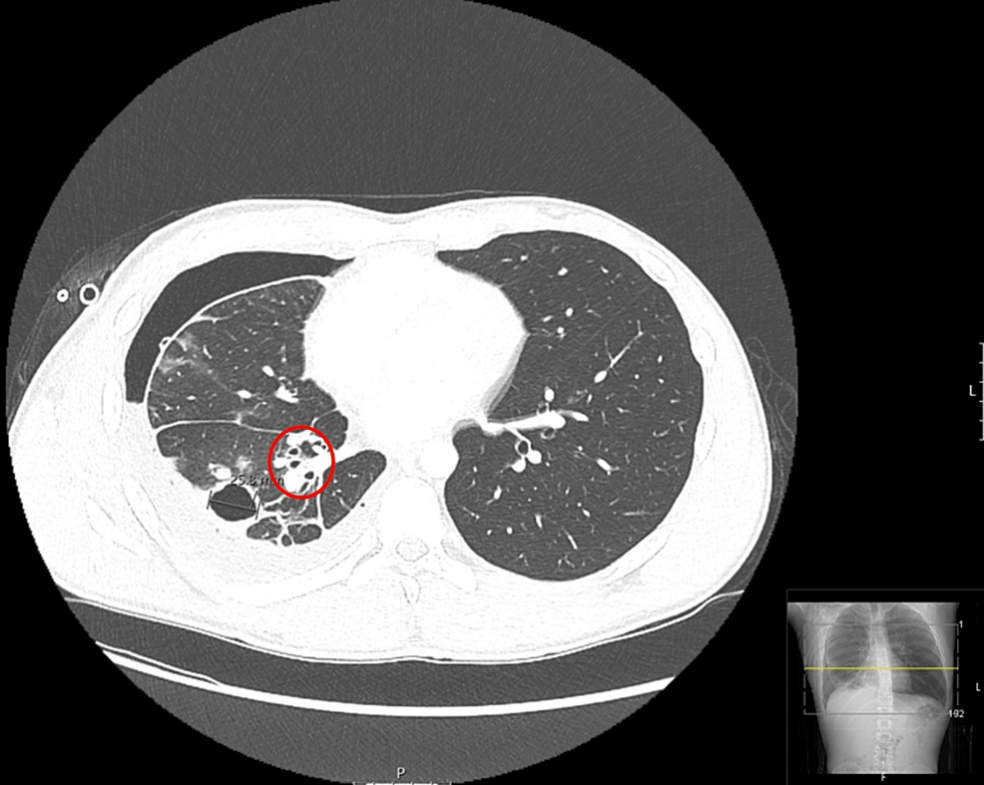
Chest CT with contrast of the lower lungs 11 days after initial CT Seen is a cystic lesion in the right lower lobe measuring 2.6 cm and slightly increased right pleural effusion with associated atelectasis. Mildly enlarged right hilar lymph nodes are also indicated (red circle).
